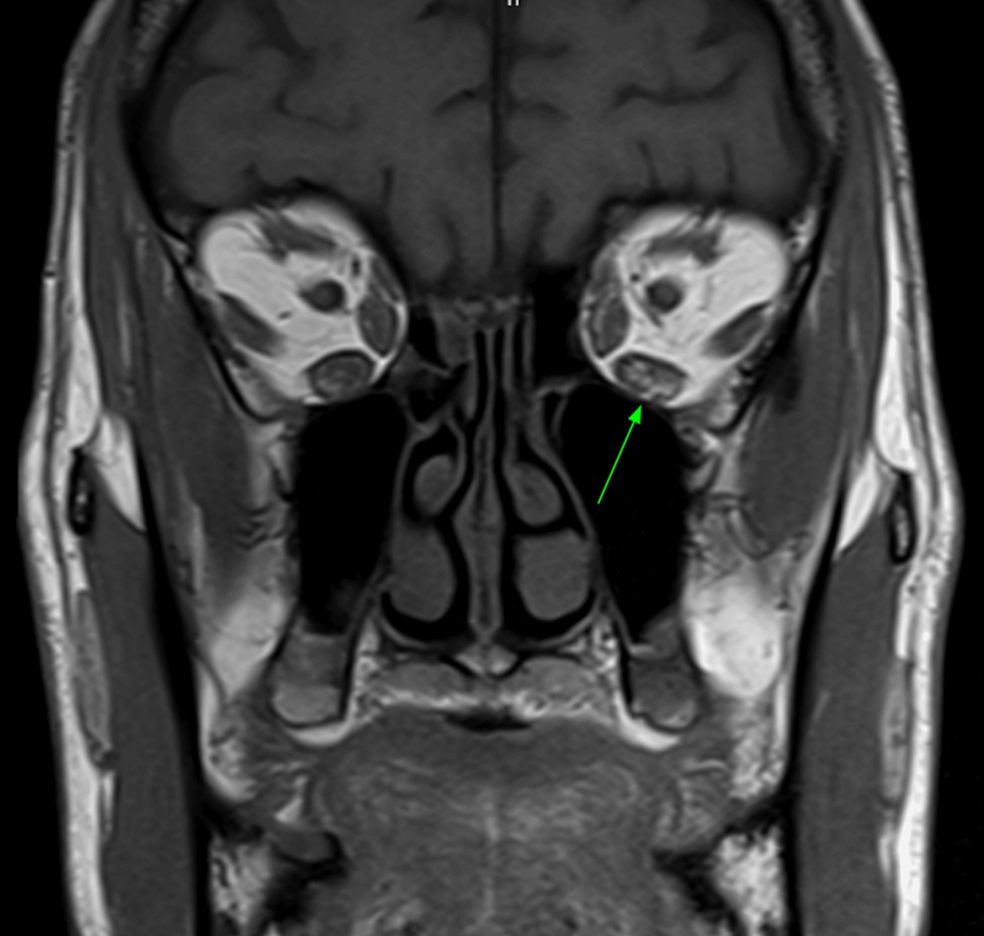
MRI, T1-weighted image, showing bilateral fatty infiltration of the inferior recti muscles, more prominent in the left eye (green arrow). Marginal intraocular muscle enlargement can also be seen in the bilateral inferior recti and lateral recti muscles.
